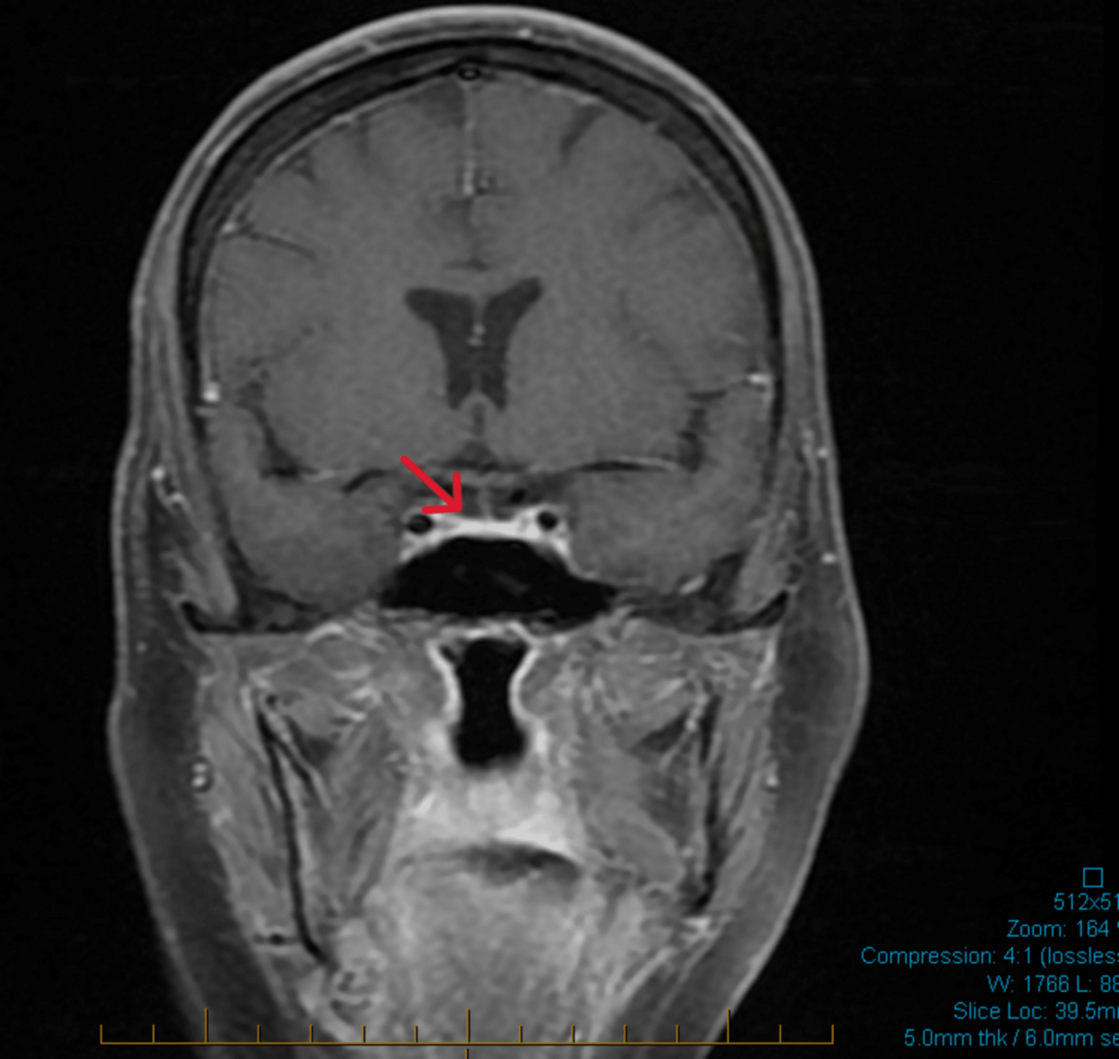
A coronal brain MRI T1-weighted image showing a normal pituitary gland, which suggests that the gland has a typical size, shape, and signal intensity without evidence of structural abnormalities (red arrow) MRI: magnetic resonance imaging
